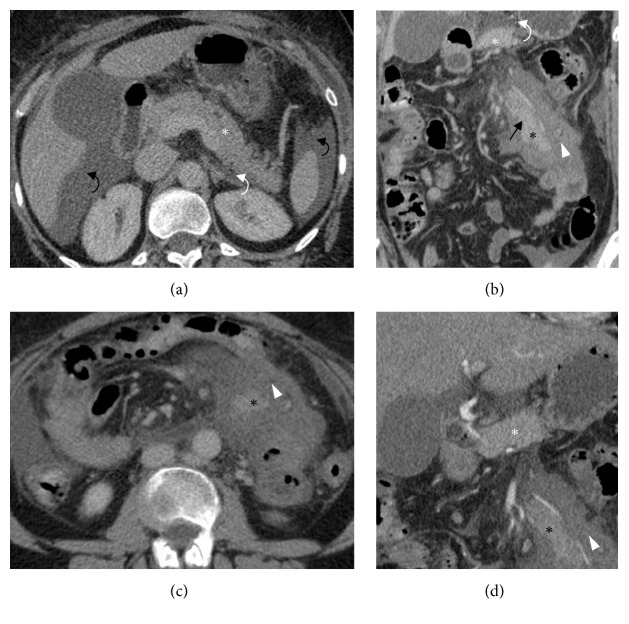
CT scan performed at the third day of admission. (a) Axial CT image in the portal venous phase. Normal appearance of the orthotopic pancreas* (white asterisks)*. A nonsignificant small amount of retroperitoneal fluid on the level of the pancreas tail* (white curved arrows)*. Presence of a large amount of free intraperitoneal fluid* (black curved arrows)*. (b-c) Coronal and axial CT image in the portal venous phase. Enhancing soft tissue mass* (black asterisk) *with surrounding mesenteric edema* (white arrowhead)*. Suggestion of a central ductal structure* (black arrow)*. (d) Coronal CT image in the early arterial phase shows a comparable enhancement of the orthotopic and heterotopic pancreas* (resp., white and black asterisks)*.
